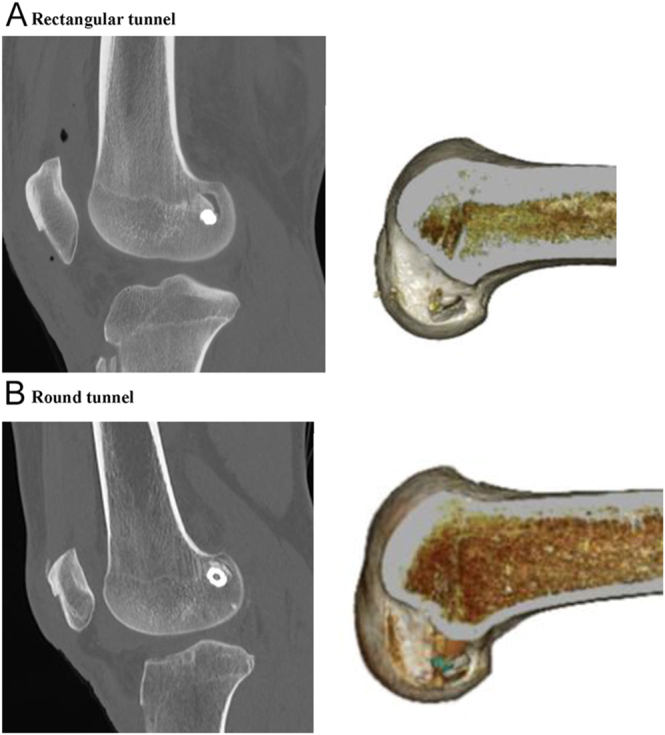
CT images with BTB fixed by interference screw: (A) rectangular tunnel and (B) round tunnel.
